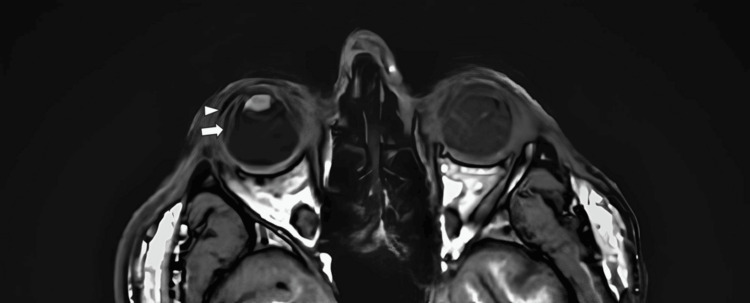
T1 MRI of the eye. Right eye: presence of fluid in the subretinal (arrow) and suprachoroidal (arrowhead) space. Left eye: Note the state of the left eye due to acute postoperative endophthalmitis.
